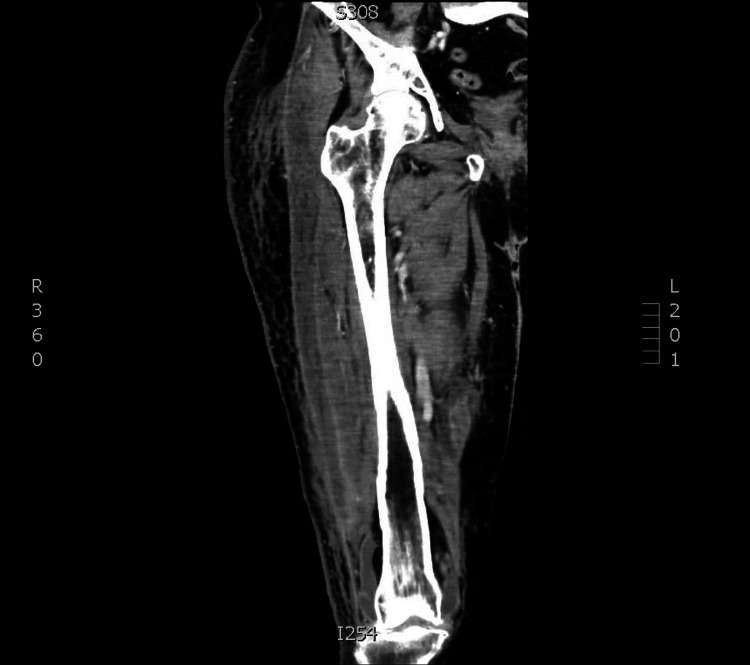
CT of the right femur #2 The rim-enhancing lesion extends the entire iliotibial band in the coronal view CT of the right femur #2 with IV contrast. Subcutaneous stranding indicative of cellulitis is also noticeable on the entire lateral aspect of the right femur.
